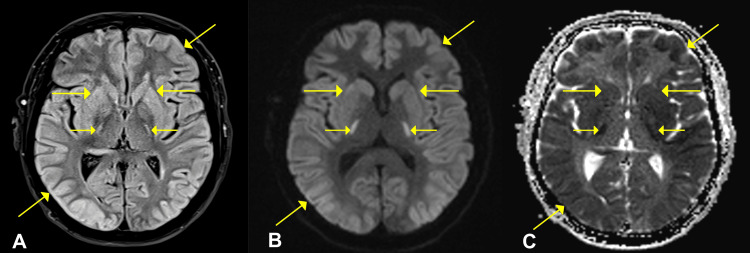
Axial brain MRI showing diffuse cortical and striatal abnormalities: (A) FLAIR demonstrating bilateral cortical hyperintensity with involvement of the neostriatum and posterior limbs of the internal capsules, (B) DWI showing symmetric diffusion restriction involving the cortex, neostriatum, and posterior limbs of the internal capsules, and (C) ADC map confirming corresponding diffusion restriction, consistent with severe hypoglycemic encephalopathy. DWI: diffusion-weighted imaging; FLAIR: fluid-attenuated inversion recovery; ADC: apparent diffusion coefficient
